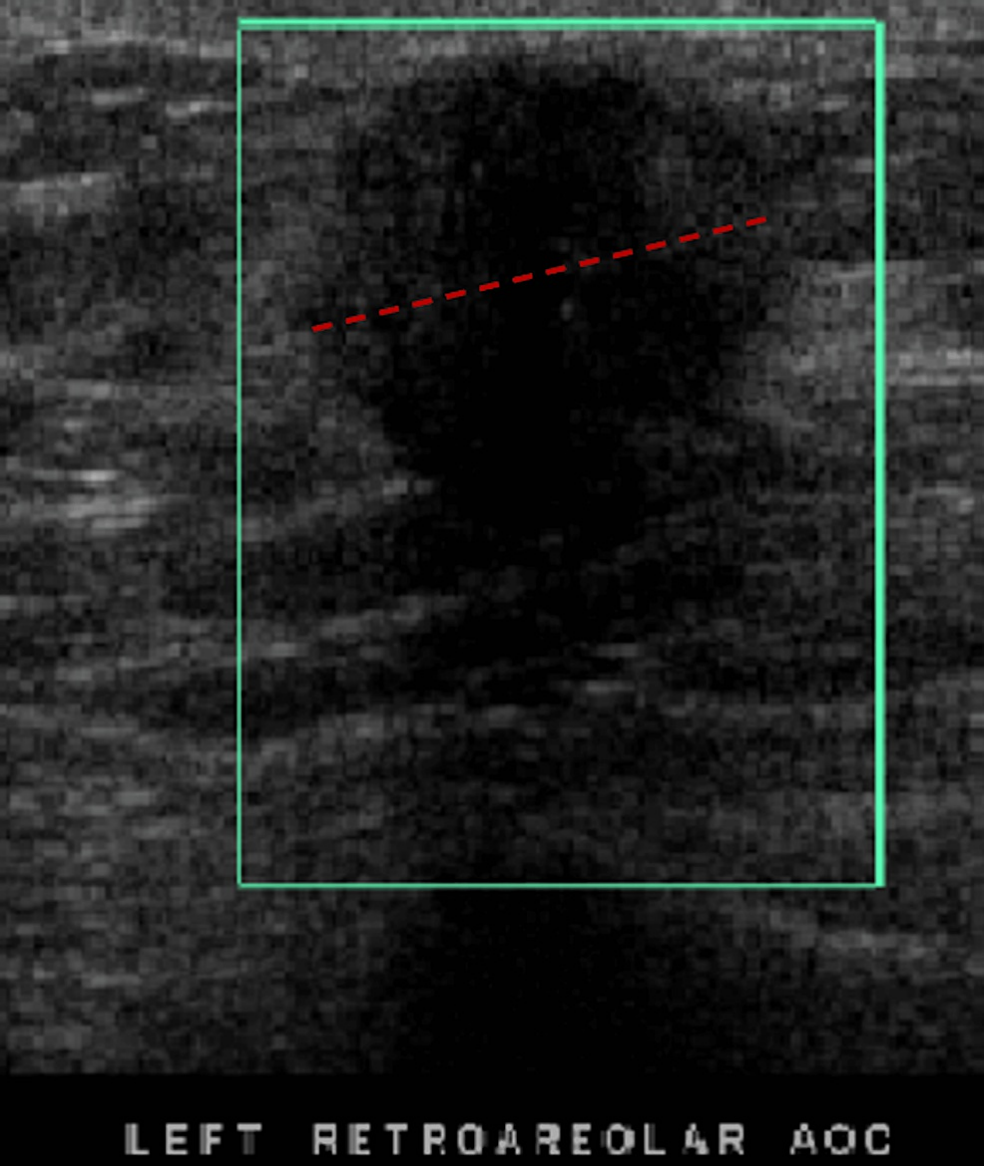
Ultrasound of the left retroareolar mass
